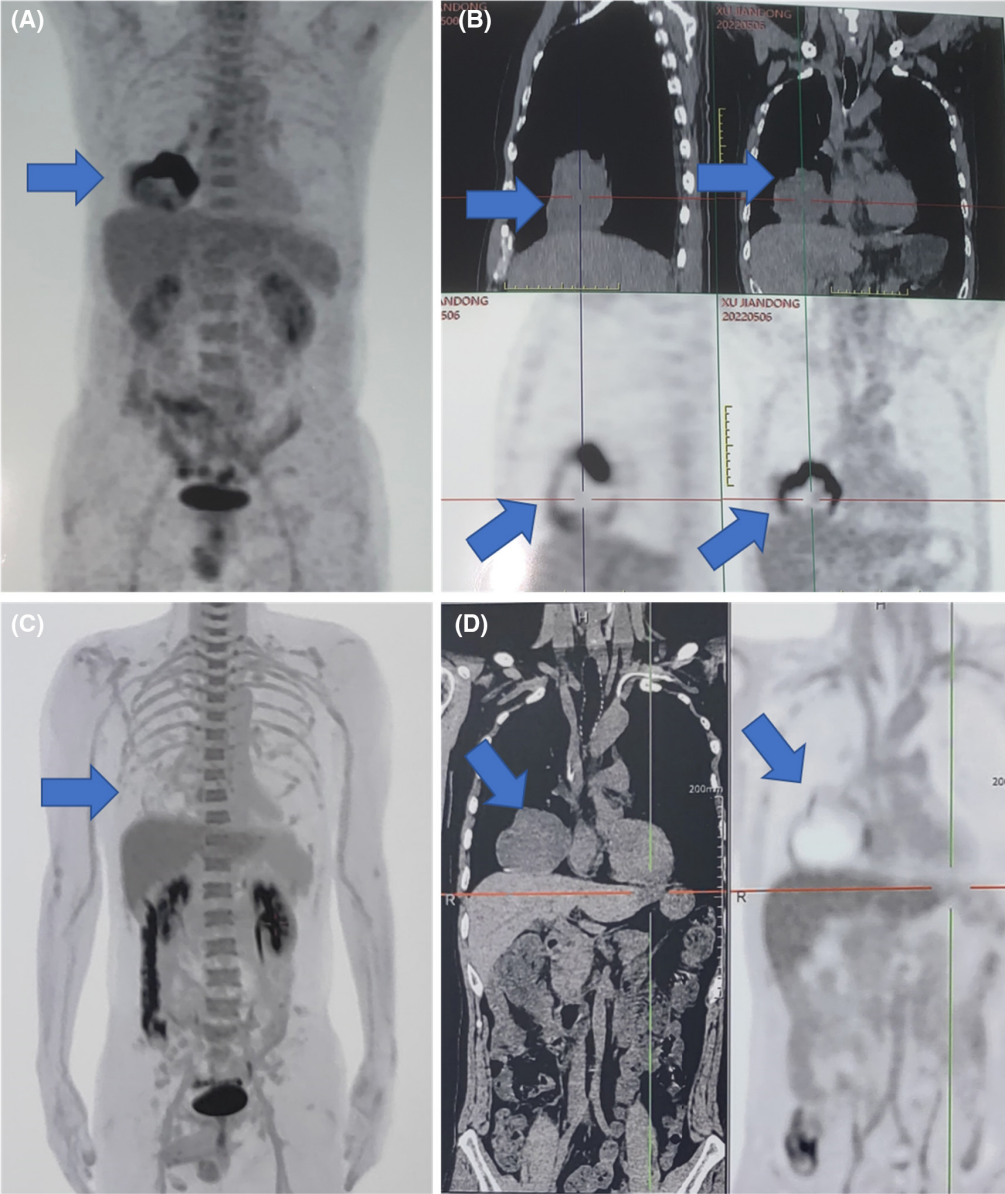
The features of PET‐CT corresponding to the patient. (A,B) A 73.2 × 52.0 mm cystic solid mass was screened in the oblique fissure of the lower lobe of the right lung. The boundary is clear. The average CT value is about 28.2 HU. The margin is accompanied by abnormal increase in glucose metabolism. The maximum SUV value is about 3.2. (C,D) A 79.8 × 65.0 mm cystic solid mass was screened in the oblique fissure of the lower lobe of the right lung. The boundary is clear. The average CT value is about 24.8HU. The margin is accompanied by abnormal increase in glucose metabolism. The maximum SUV value is about 3.2.
